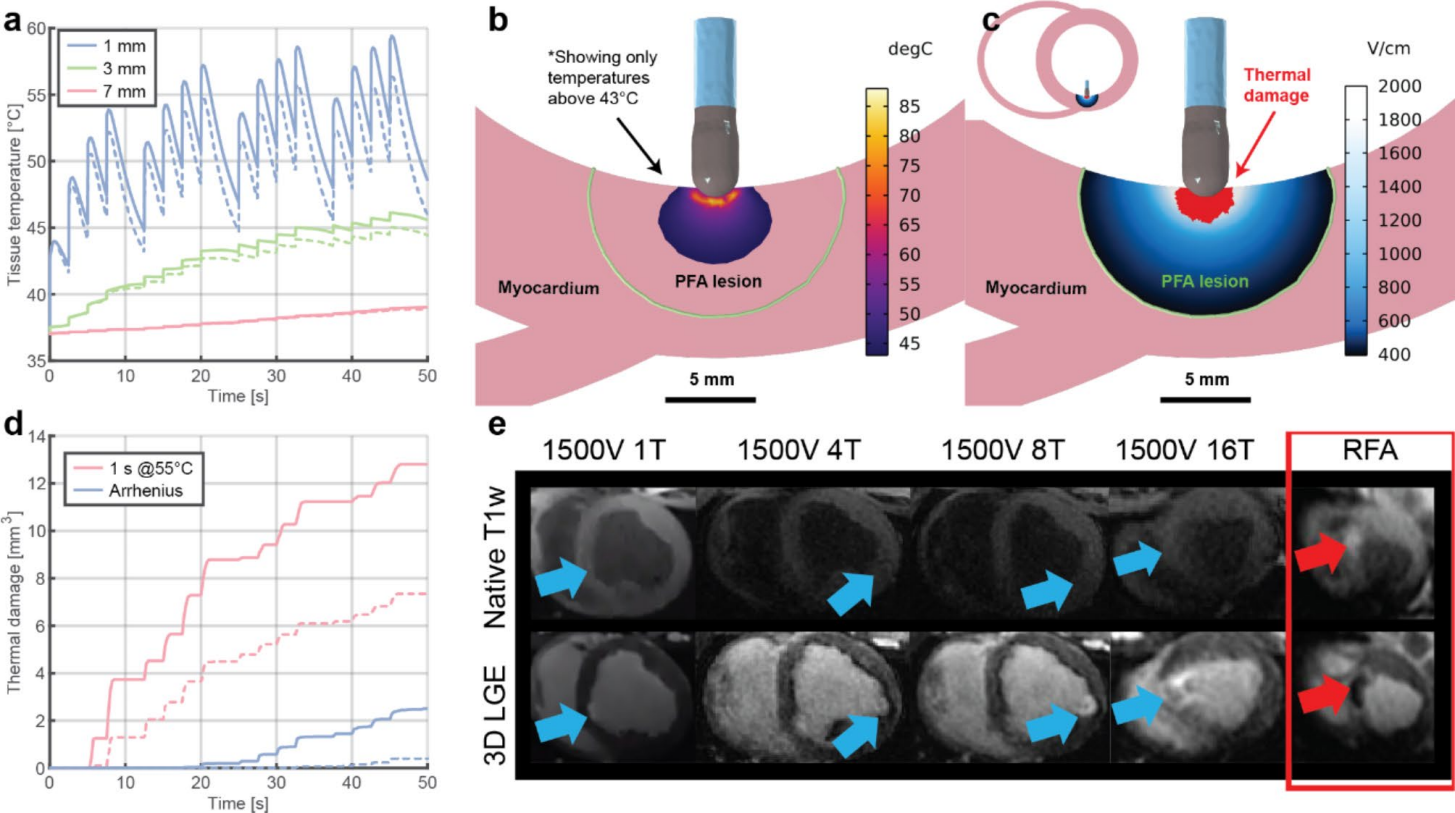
Nonthermal vs. thermal damage by Pulsed Field Ablation. (a) Calculated temperature in the myocardium at depths of 1, 3 and 7 mm for the 16-pulse-train protocol. Solid lines indicate low blood flow scenario and dashed lines indicate high flow scenario. (b) Calculated temperature distribution in the myocardium at the end of the 16th train (peak temperature). Only temperatures above 43 °C are shown. Black line indicates possible size of the PFA lesion based on the 16-train 7-day MRI LET (median value: 394 V/cm, Fig. 6b). Grid size is 1 × 1 mm. (c) Predicted nonthermal vs. thermal damage for 16-train protocol. Calculated electric field distribution in the myocardium indicates the PFA lesion based on the 16-train 7-day MRI LET – only electric field values above 394 V/cm are shown. Red area indicates possible thermal damage using a threshold of 1 s at ≥ 55 °C in low blood-flow condition (worst case). (d) Calculated thermal damage for 16 train protocol using the threshold of 1 s at ≥ 55 °C and Arrhenius integral - probability of cell death exceeding 63%. Solid lines indicate low blood flow scenario and dashed lines indicate high flow scenario. All temperature and thermal damage calculations shown here were done for the mid posterior lesion, for which the model predicts the highest electric current. (e) Native T1w and 3D LGE MRI at different PFA doses at 24 h post-ablation (blue arrows), in comparison to same acquisitions for RFA (red arrows and red frame). Native T1w PFA images show absence of hyperintensity at lesion locations suggesting lethal thermal threshold was not achieved, compared with clear hyperintense lesion in RFA indicating lethal thermal damage. 3D LGE cMRI demonstrates hyperintensity in PFA locations despite the absence of hyperintensity in native T1w with some microvascular obstruction (MVO) structure at highest PFA dose, while image of RFA lesion shows large MVO. RFA – Radiofrequency ablation; LGE – Late gadolinium enhancement; PFA - Pulsed field ablation; T- train.
